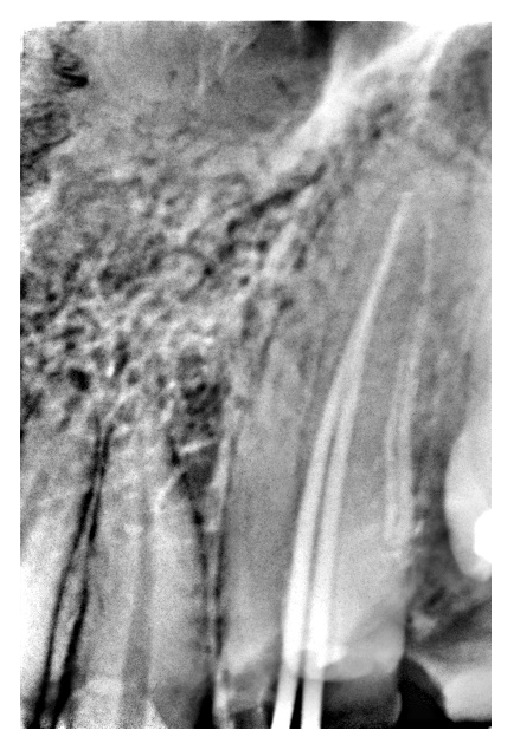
Radiograph showing master cone gutta-percha.
